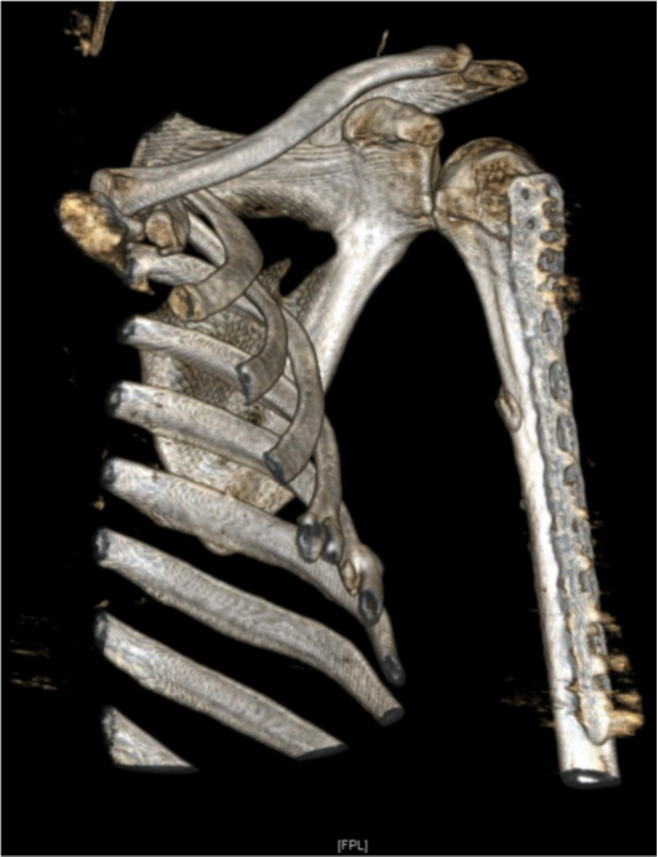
Computed tomography scan showing radiological union of scapular and humeral fracture.
